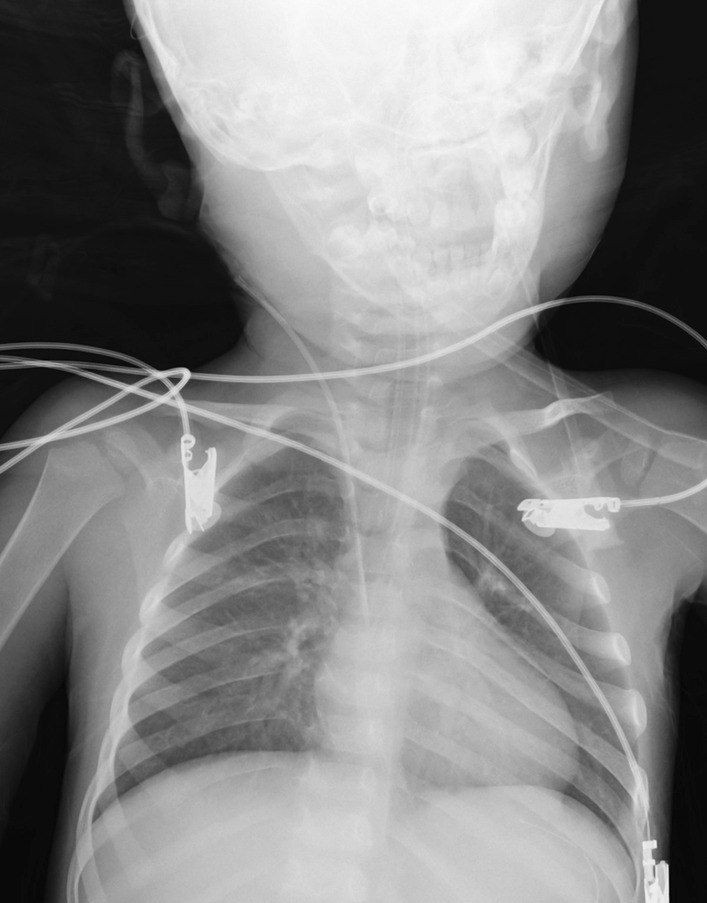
A chest X-ray shows bilateral upper zone opacities and right hilar shadow
